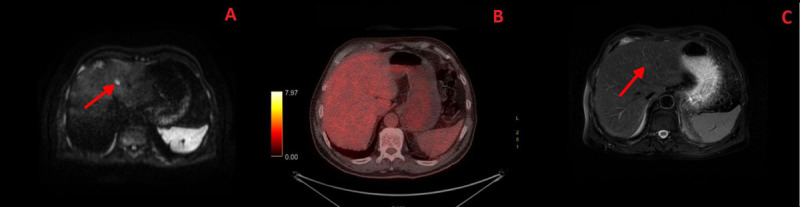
Axial plane; A- Diffusion-weighted MRI image with a b value of 1000; B- Fused PET-CT image; C- T2-weighted MRI.
